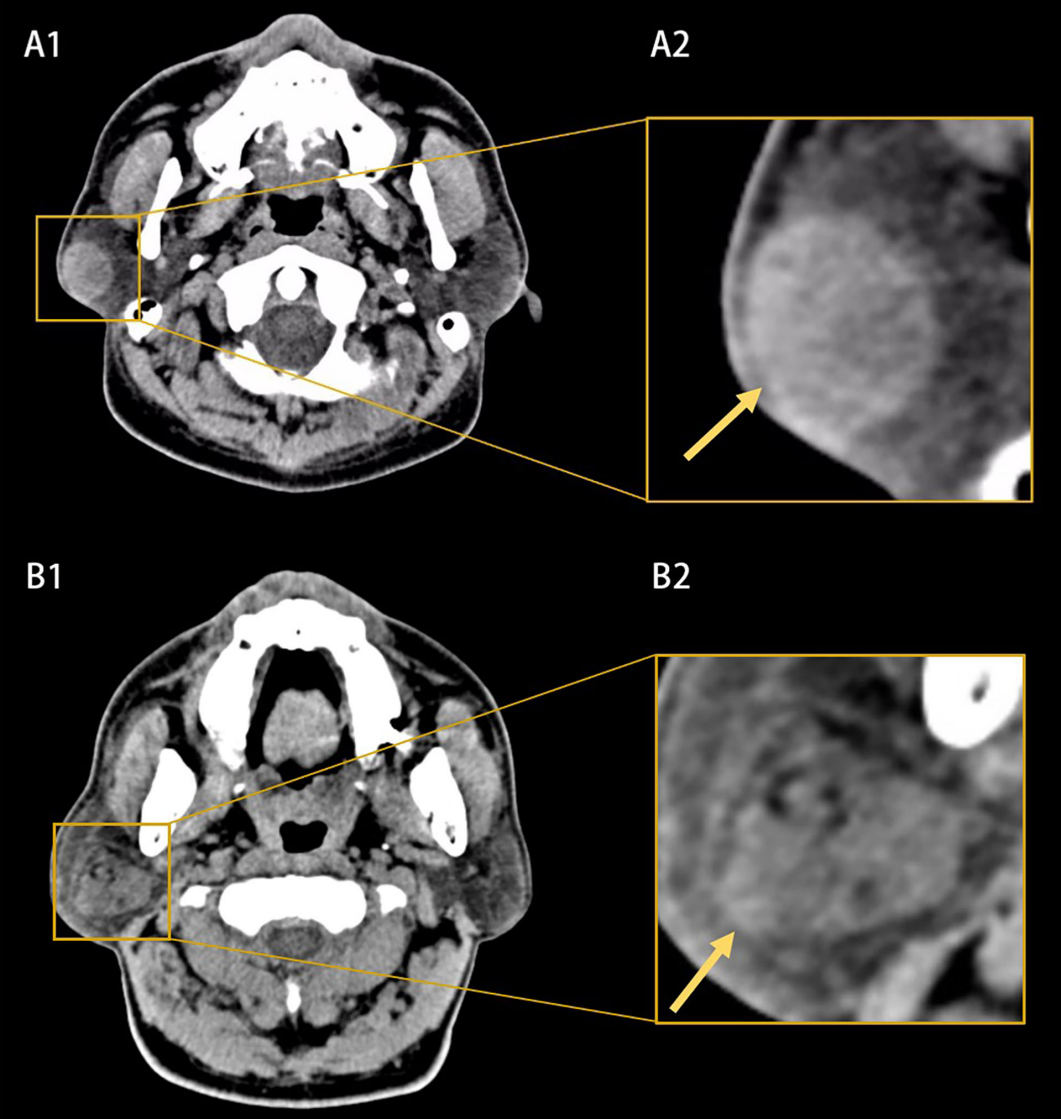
Example of computed tomography (CT) images (A1, B1) and the extracted region of interests (A2, B2). (A1, A2) Showed a benign parotid tumor with a well-defined and smooth border and homogeneous appearance (yellow arrow). (B1, B2) Showed a malignant parotid tumor with a poor-defined border and heterogeneous appearance (yellow arrow).
